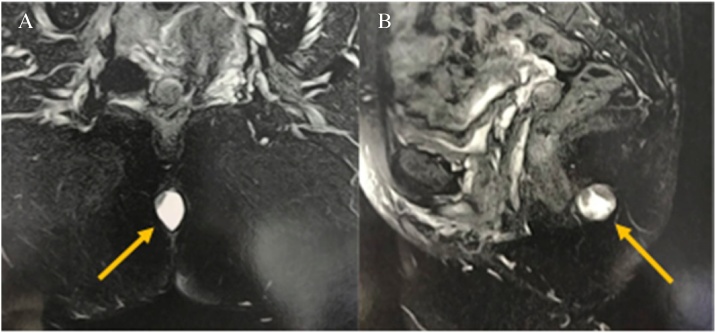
(A, B) Magnetic resonance imaging of the pélvis with a single cystic, nodular image.
